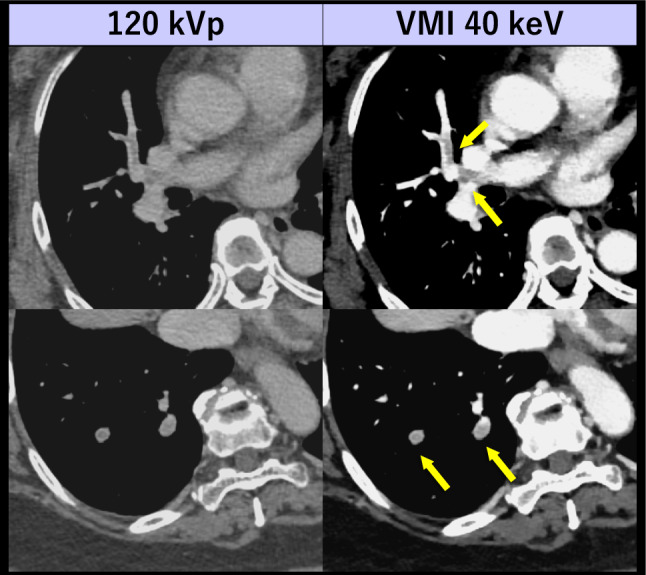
A 66-year-old woman with breast cancer who underwent portal venous phase CT using dual-layer detector scanner. Incidental PEs (arrows), which may be missed on 120 kVp images due to suboptimal contrast enhancement in the pulmonary arteries, are confidently detectable on VMI 40 keV without the need for dedicated CTPA
